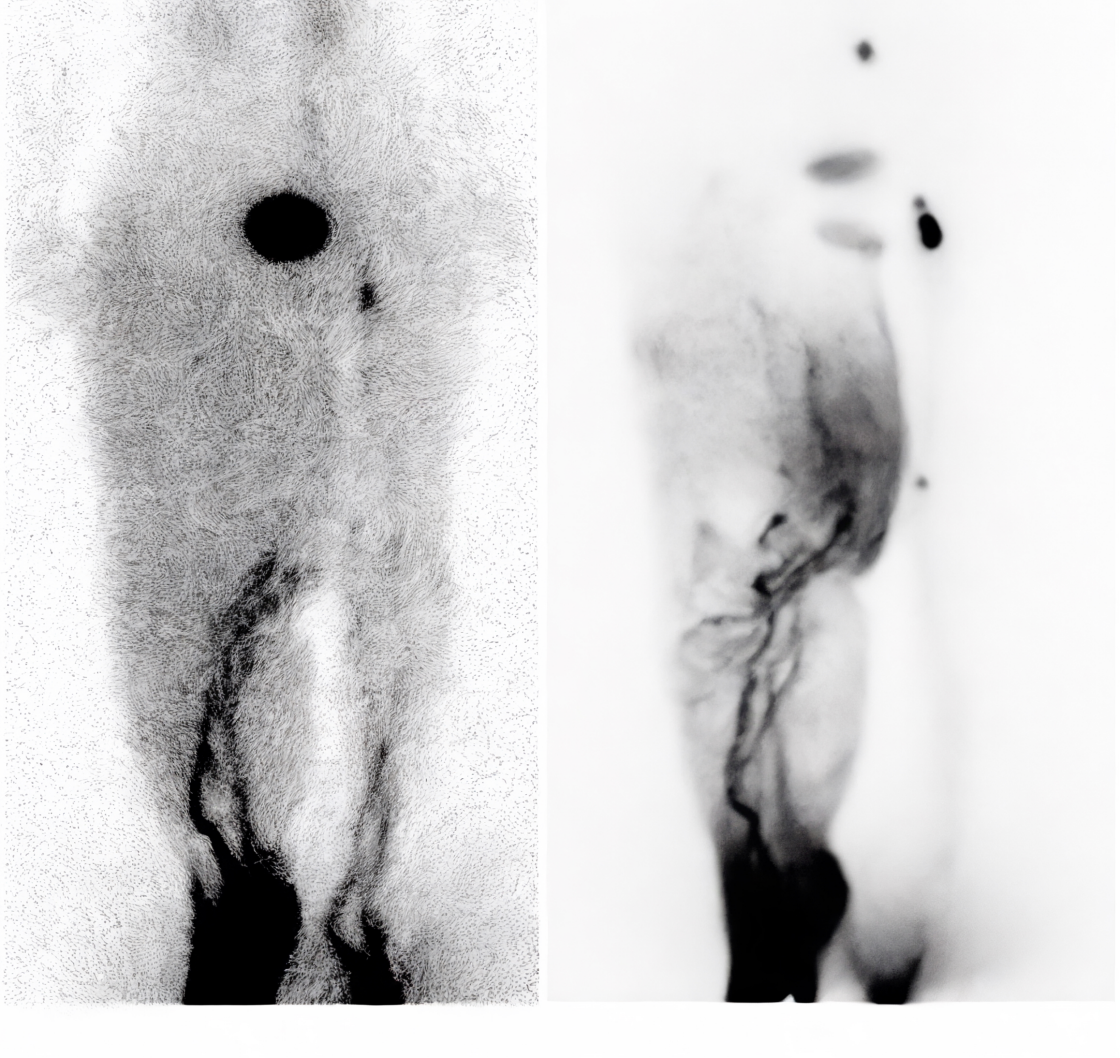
(A) Preoperative lymphoscintigraphy showing dermal backflow throughout the right leg, with main lymphatic vessels visible only below knee level. (B) Lymphoscintigraphy one year postoperatively showing significant improvement in lymphatic drainage. Main lymphatic vessels were visible above knee level, with reduced dermal backflow.
